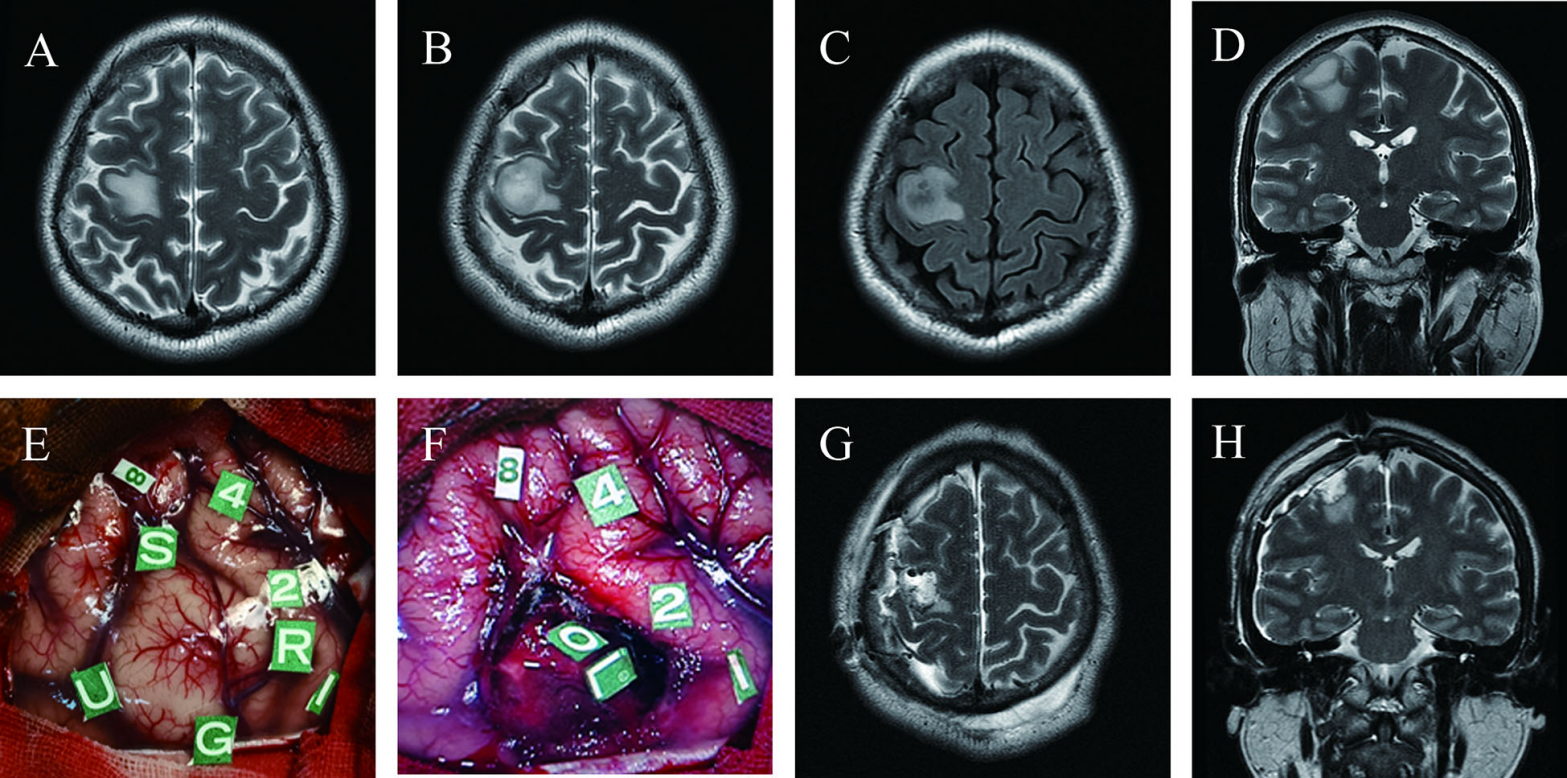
Maximum safe resection of astrocytoma with IDH mutation (WHO Grade 3) in the hand knob of the right precentral gyrus under awake craniotomy in a 38-year-old man presenting with seizures (Case 11); Pre-operative T2 axial (A, B), T2 FLAIR axial (C) and T2 coronal (D) MRI revealed the tumor’s location precisely on the hand knob of the right precentral gyrus; (E) Intraoperative view before tumor resection; Tumor borders marked with letter tags (Tag U, anterior; G, superior; S, inferior; R, posterior). Number tags show positive points of DES mapping (8, changes in vocal tone; 1, 2, 4, primary sensory cortex, paresthesia of the left forearm, palm, and thumb, respectively); (F) Intraoperative view subsequent to tumor removal; Tag L, subcortical area for wrist movement; O, subcortical area for finger movement; Postoperative axial (G) and coronal (H) T2-weighted MRI demonstrated total tumor resection.
